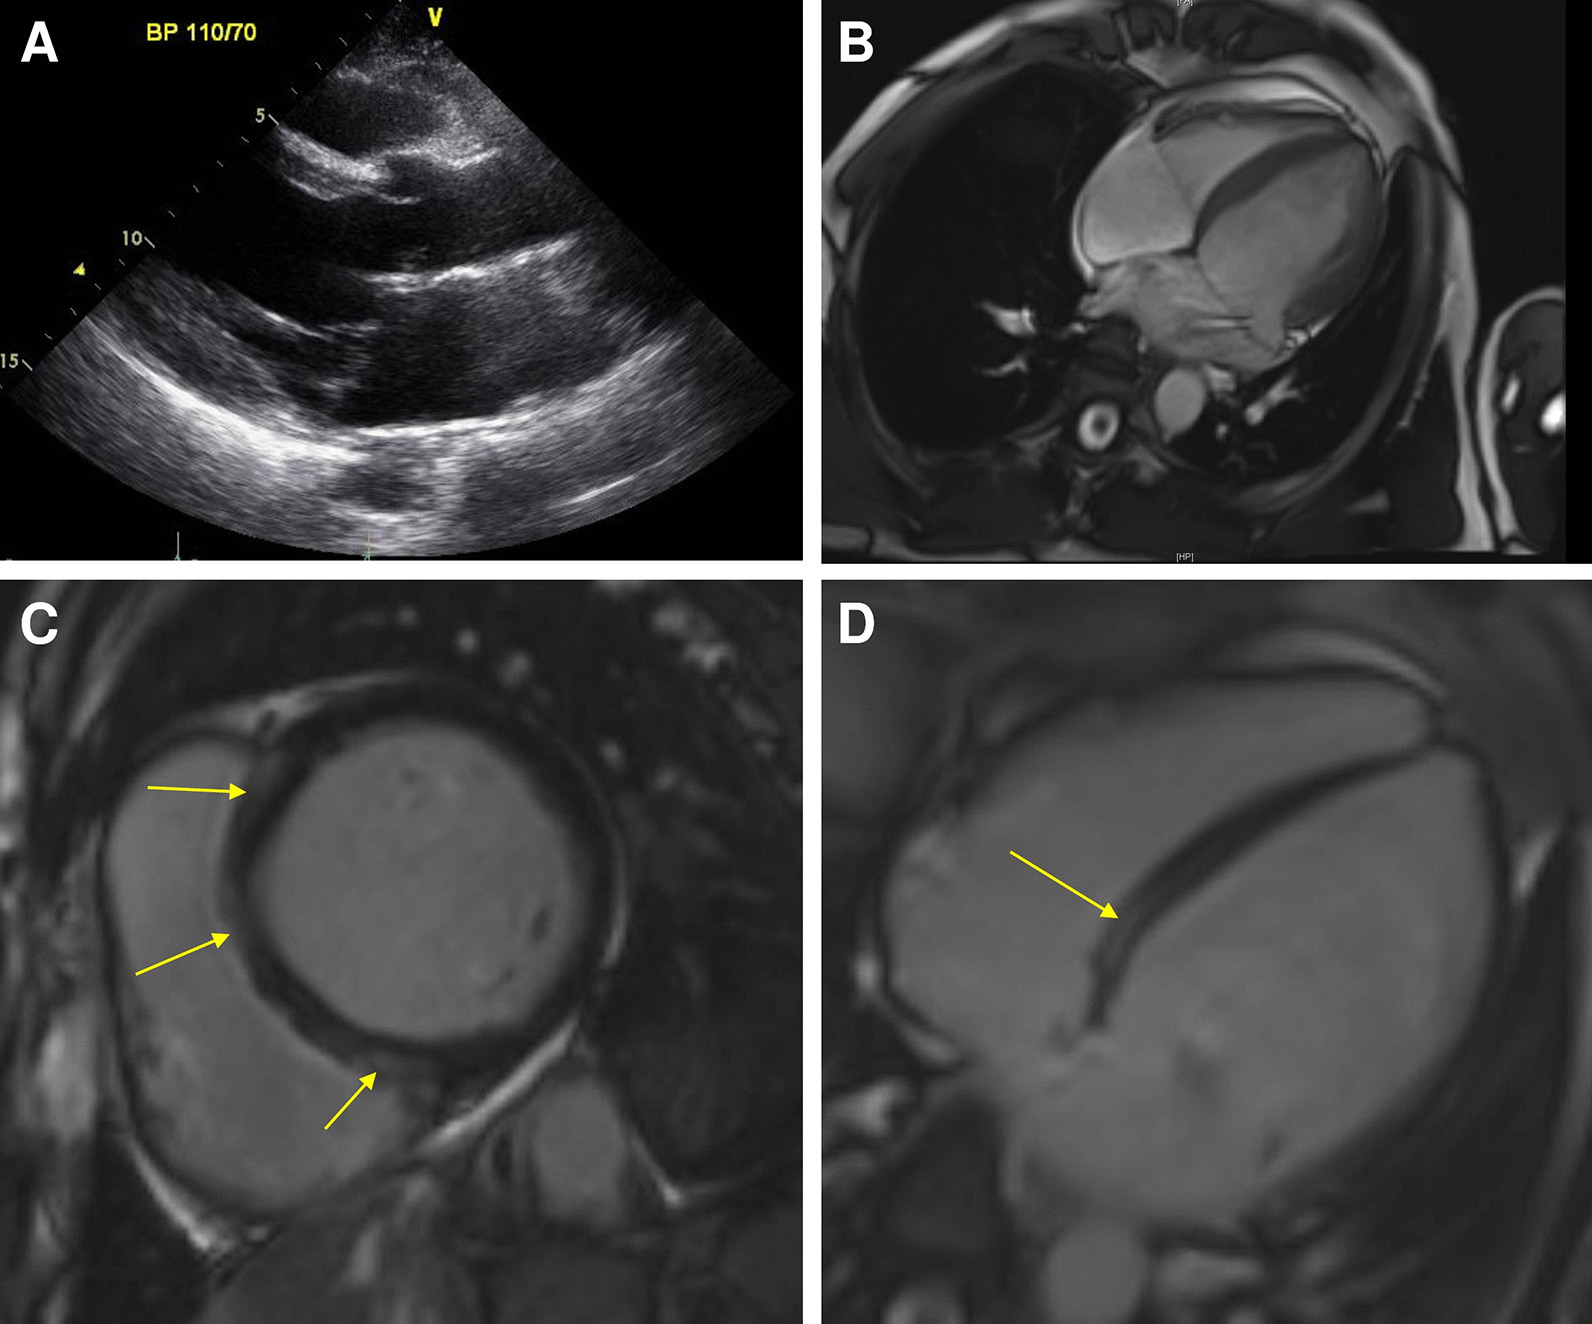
Echocardiographic and CMR findings of an MVP patient who experienced SCA. Echocardiographic image of the parasternal long axis view (A) and CMR image of 4-chamber view (B) showing prolapse of both mitral leaflets. CMR images of the short axis view (C) and 4-chamber view (D) showing LGE (arrows) at the mid-layer of the basal septum. *CMR* cardiac magnetic resonance, *LGE* late gadolinium enhancement, *MVP* mitral valve prolapse, *SCA* sudden cardiac arrest
